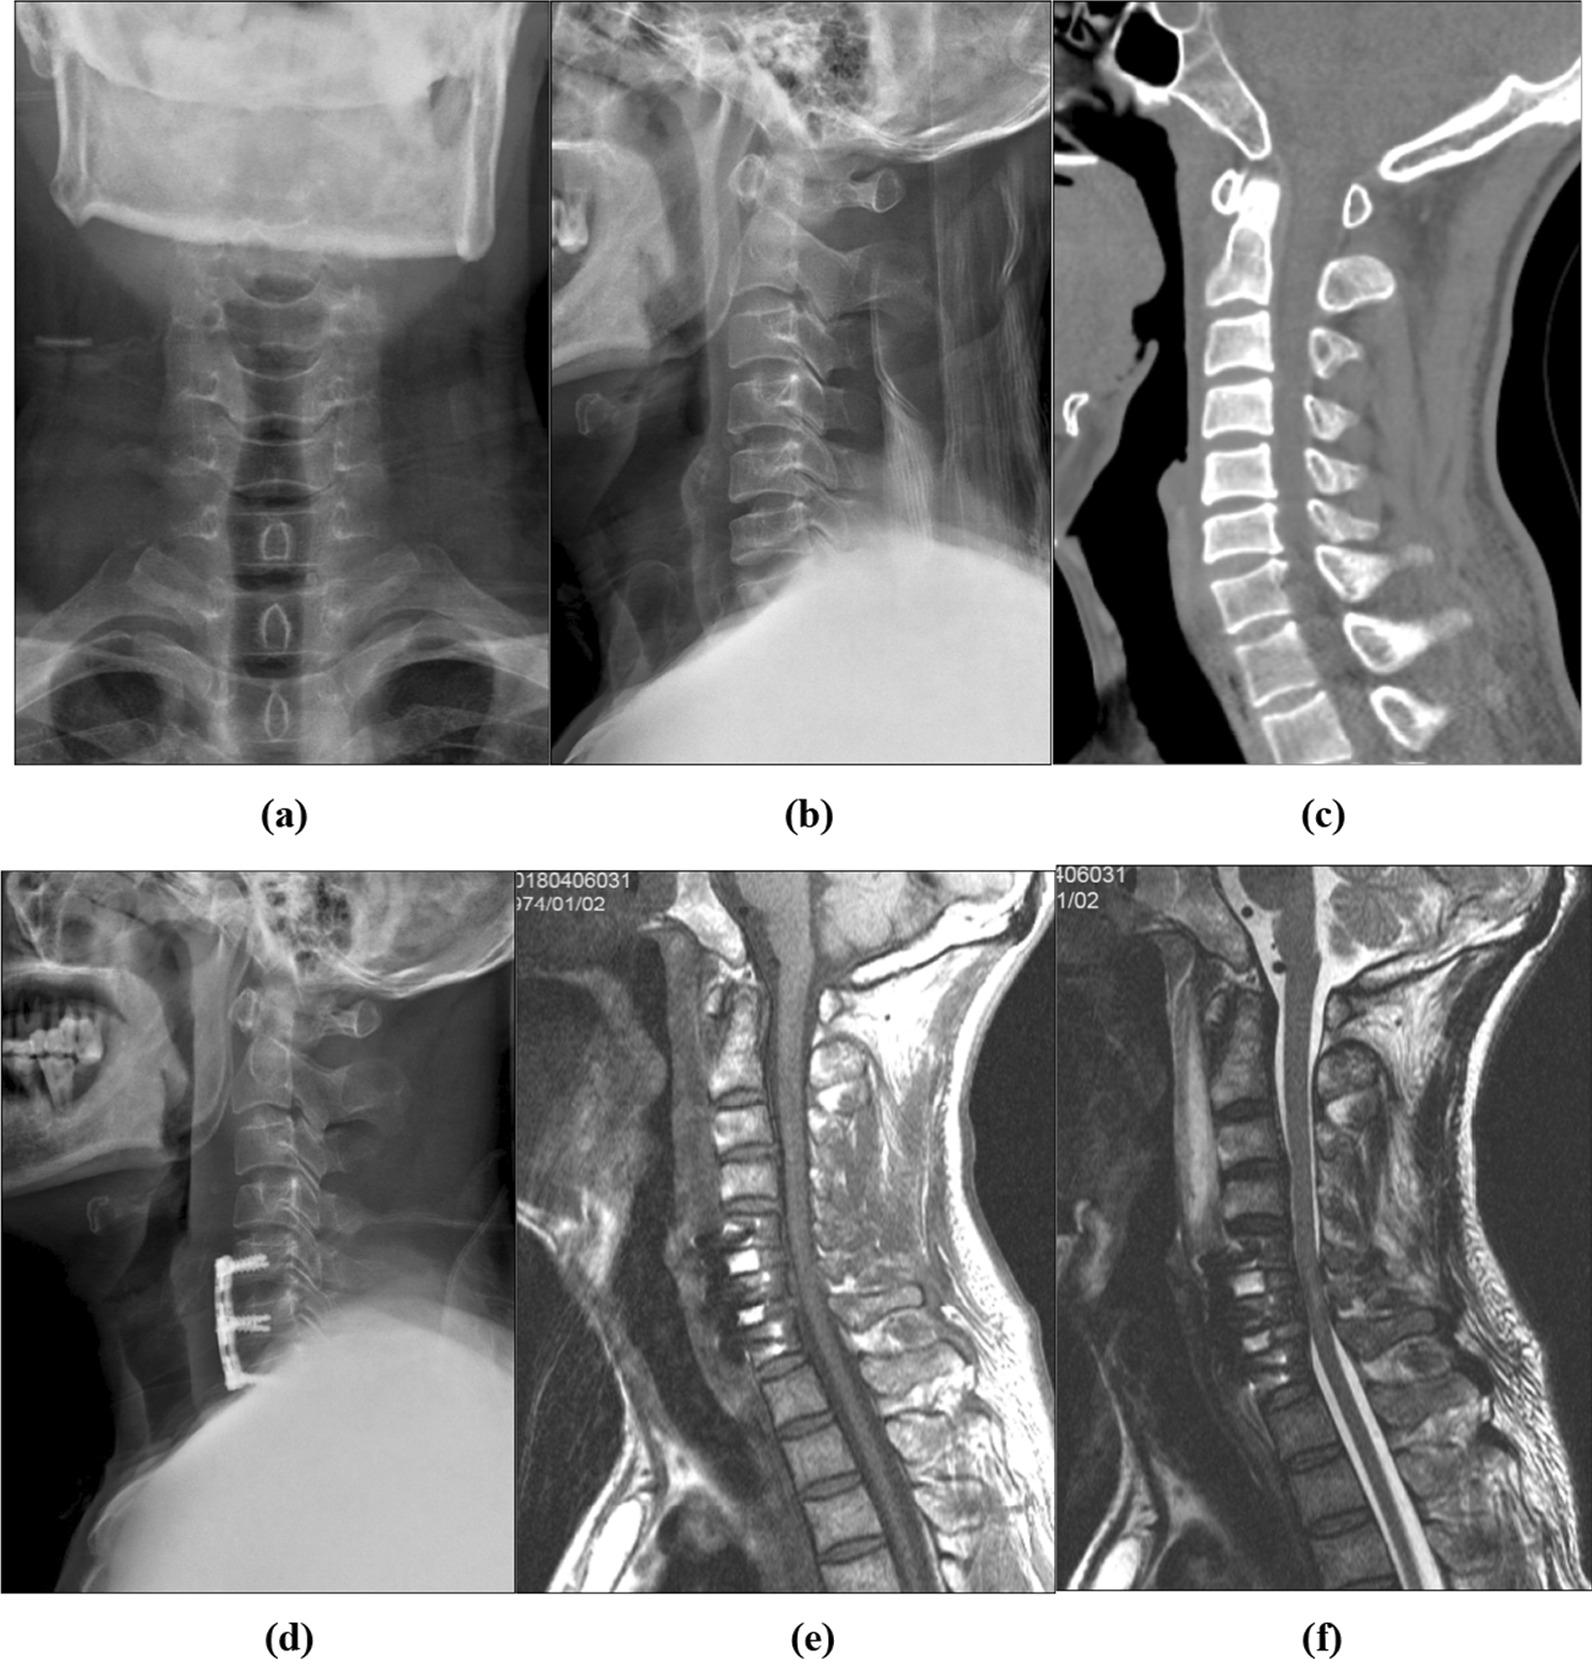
Cervical radiography (a, b) and CT (c) showed reduced cervical physiological curvature and mild hyperostosis with no distinctive fractures in a 45 year-old male patient (case 2). Post-operative reexamination by radiography (d) showed the favorable position of internal fixation and no apparent compression of the spinal cord (e, f)
